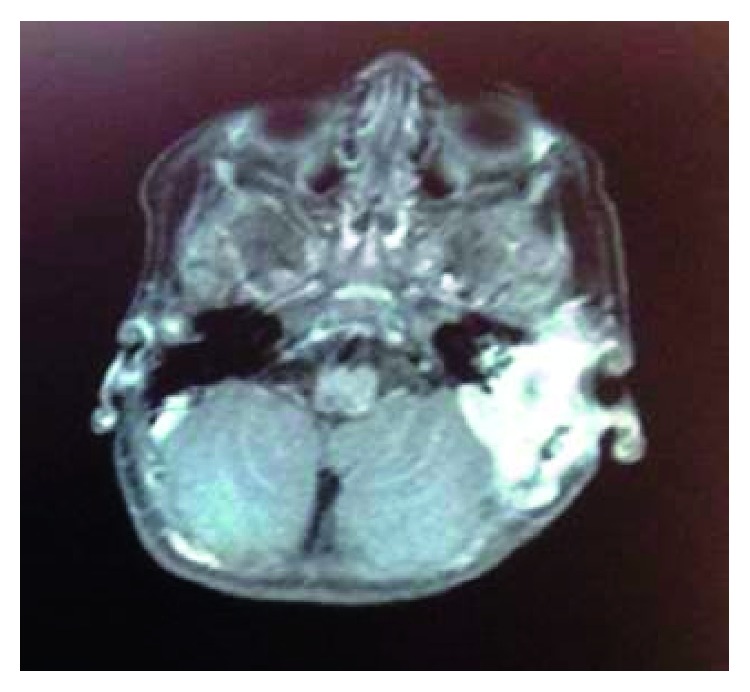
First MRI: pathological magnetic signal with mild enhancement by contrast media and areas of necrosis of all cavities of the temporal bone. Whether this lesion corresponds to a residual disease or postoperative lesions remains a question
